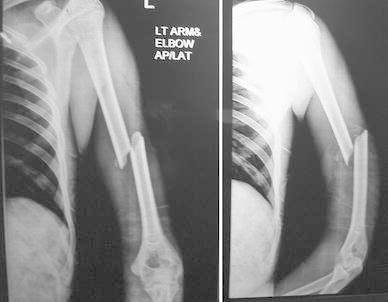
AP and lateral radiographs of a fracture shaft of humerus in the middle third
